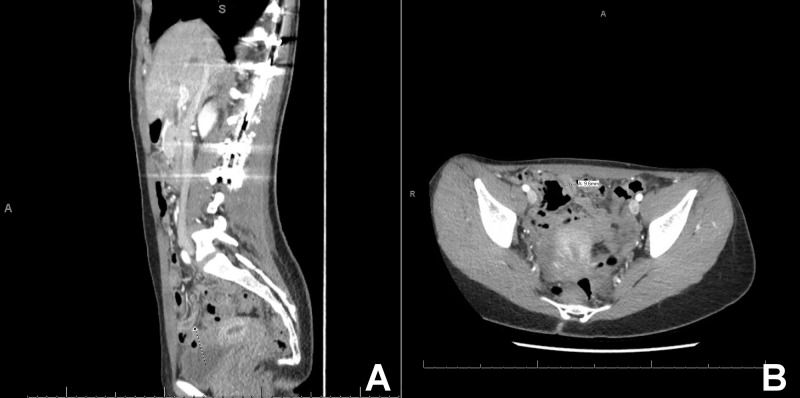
Contrast-enhanced computed tomography (CT) scan Select sagittal (A) and axial (B) contrast-enhanced CT images demonstrating a hyperenhancing thick-walled tubular structure in the right lower quadrant/pelvis, likely representing an inflamed appendix
